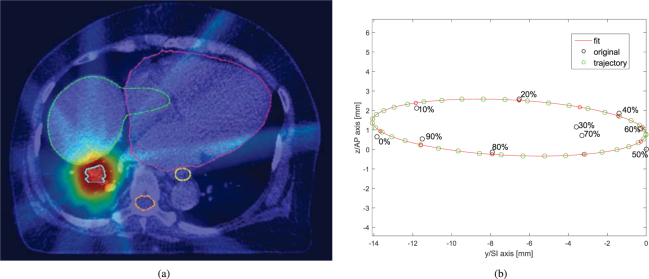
Lung patient case used to assess the performance of our EMT implementations. (a) A transversal slice of CT is shown with a dose wash overlay characterising the accumulated dose. The contours represent local anatomy: eusophagus (yellow), spinal cord (orange), liver (green) and heart (red). (b) motion trajectory of the tumour target.
